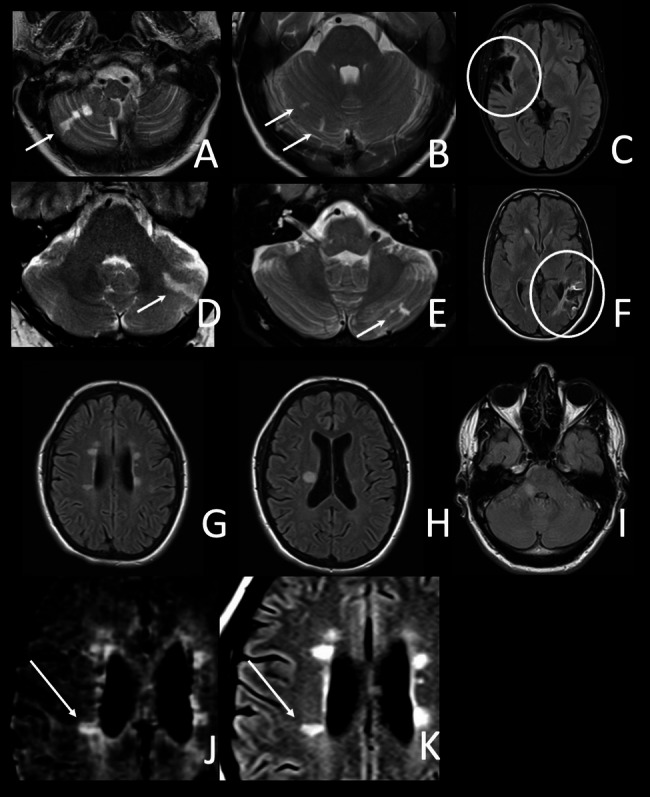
Postischemic and inflammatory lesions in MRI. (A-F) emboliform infarctions. A, B,D, and E show cerebellar infarctions, C and F show middle cerebral artery infarctions. (G and H) show periventricular, ovaloid lesions (Dawson’s fingers) of one patient. I) shows a singular lesion in the right cerebellar peduncule, (J) shows a central vein of a periventricular lesion on susceptibility weighted imaging (SWI). (K) depicts the same lesion as J on a fluid-attenuated inversion recovery (FLAIR) image. The patient shown in G, H, J and K was diagnosed with and treated for both Fabry disease and multiple sclerosis
